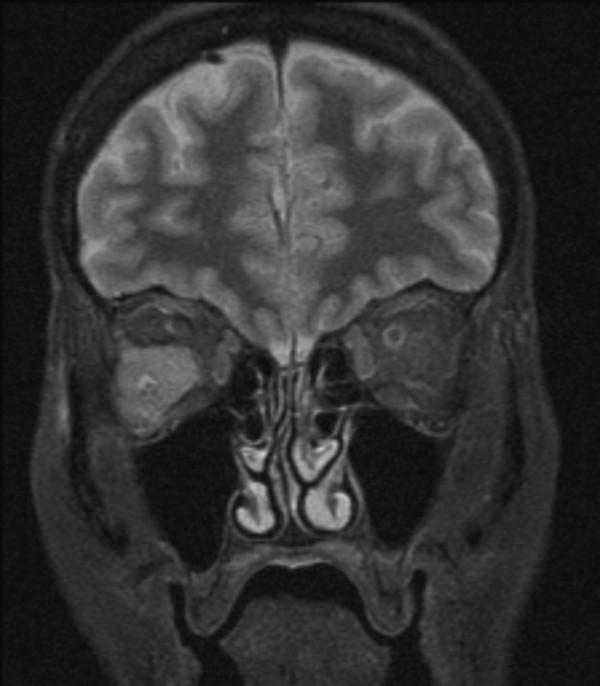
Coronal T1 MRI brain and orbit. MRI performed 2 weeks after fine needle aspiration biopsy of the right orbital mass, but prior to commencing chemotherapy. The right orbital mass is shown causing displacement of the optic nerve.
